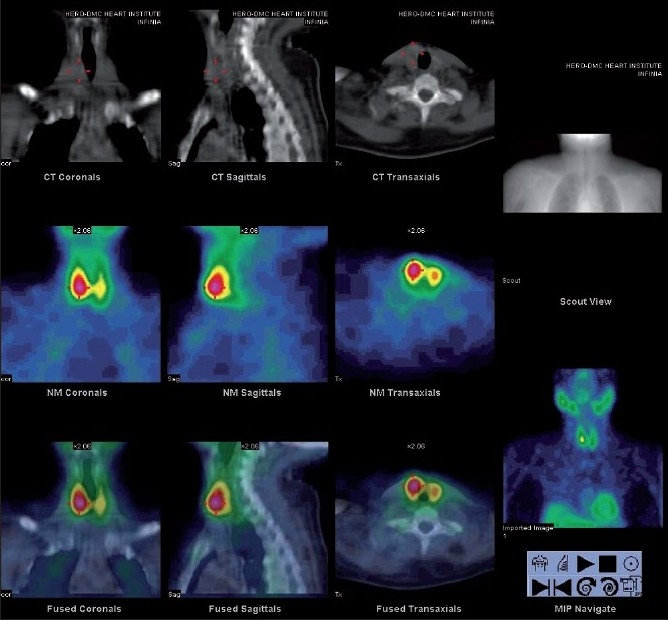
Intrathyroidal parathyroid adenoma in 46 years old woman of primary hyperparathyroidism. Coronal, sagittal and axial delayed-phase fused SPECT/CT images depict a focus of delayed washout at the level of the lower pole of the right thyroid lobe
